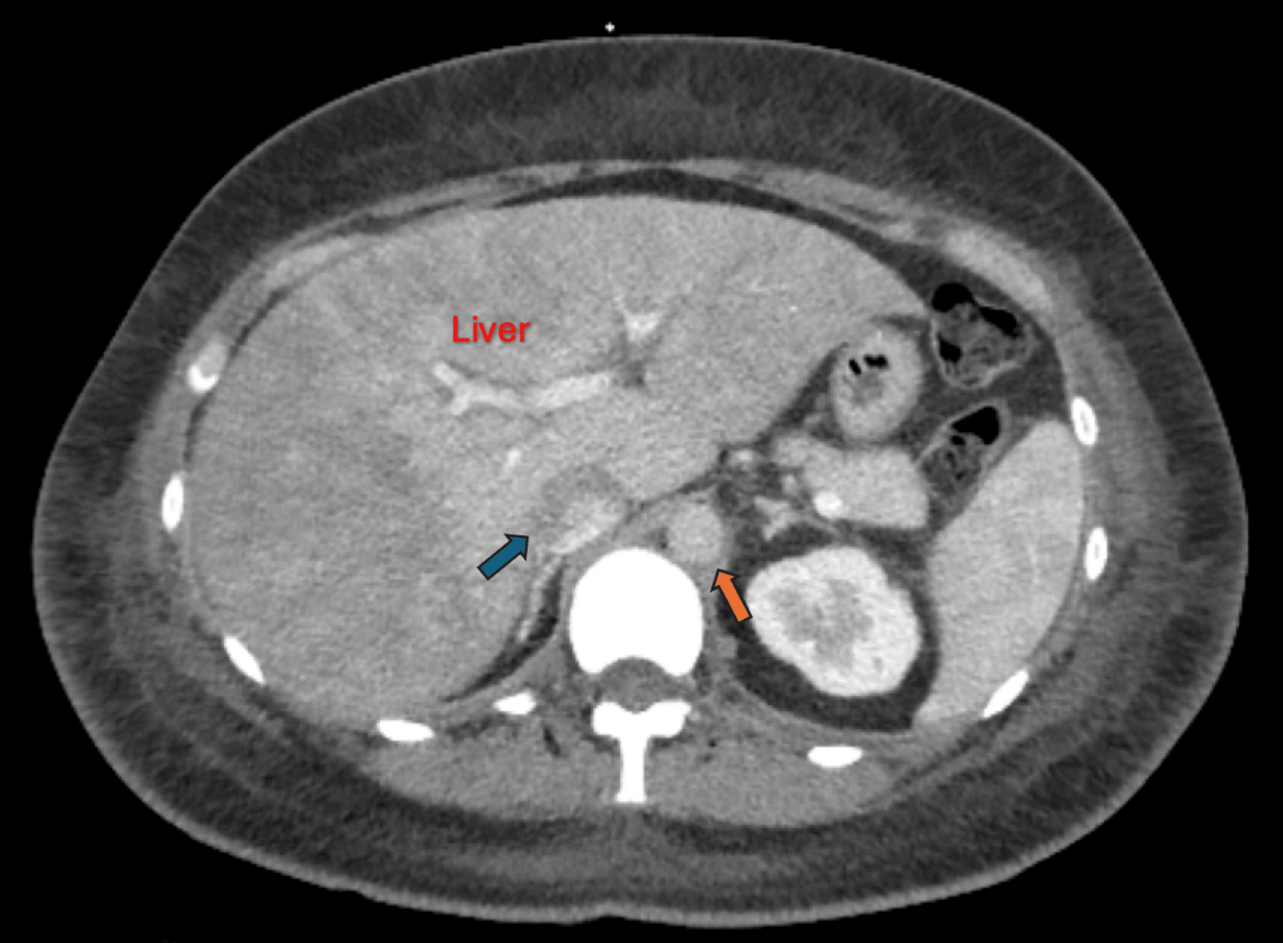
Computerized tomography (CT) of the abdomen with contrast (axial cut) at the level of the upper abdomen The image shows congestive hepatomegaly, with the blue arrow pointing to the distended inferior vena cava. Together, this indicates elevated right heart pressures. For reference, the orange arrow marks the aorta.
